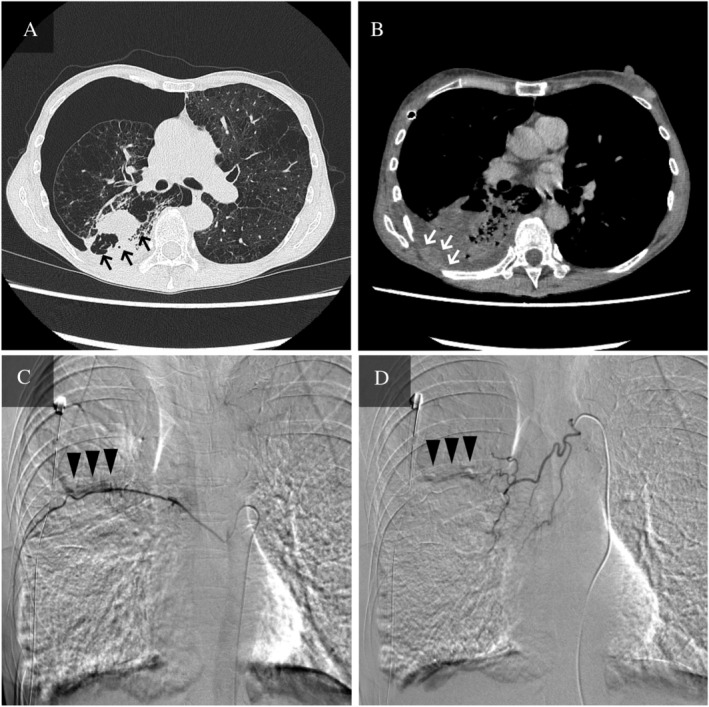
Chest computed tomography (CT) and angiographic findings. (A) CT on admission day revealed a right secondary pneumothorax of emphysema. There was a pleura‐attached mass shadow with cavity at the periphery of the right lower lobe (S6); the diameter was 35 mm (black arrows). There was partial thickness of the parietal pleura and no pleural effusion. (B) CT with contrast agent on the day of haemothorax and haemoptysis. There was consolidation in the right middle/lower lobes, and a right pleural effusion had appeared. There was an 18‐mm‐diameter nodule invading the chest wall at the right eighth rib level (white arrows). A chest tube was inserted via the front chest. (C) Angiography of the right eighth intercostal artery showed contrast agent in the vicinity of the pleural nodule (black arrowheads). This site was considered the origin of bleeding. (D) Angiography of the right bronchial artery revealed contrast agent in the same area as the right eighth intercostal artery (black arrowheads).
